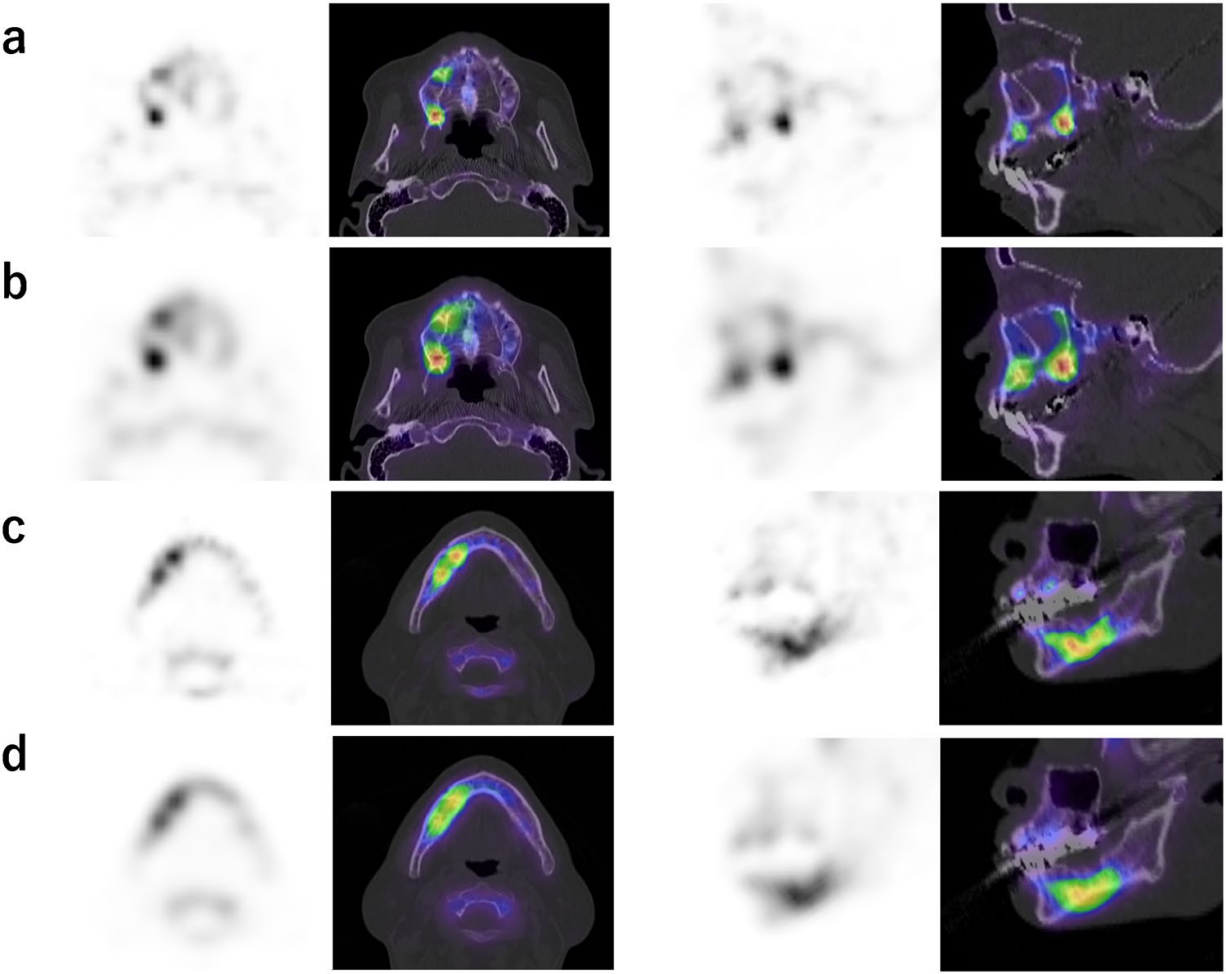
Jawbone SPECT images and fused SPECT/CT images of MRONJ of the maxilla (case 4) and mandible (case 10) using OSEM_jaw_ (a, c), OSEM_current_ (b, d). In case 4, OSEM_jaw_ clearly improved the contrast and sharpness of the lesion area. The slice presented a transaxial image along the maxilla and a sagittal image along the right maxilla. The SUV_mean_ of the lesion area was 14.8 and 7.2, respectively, and the mean visual score was 4.25 and 3.00. In case 10, OSEM_jaw_ not only clearly distinguished the lesion but also delineated the shadows within the hot area. The SUV_mean_ of the lesion area was 8.1 and 6.9, respectively, and the mean visual score was 3.75 and 3.00
